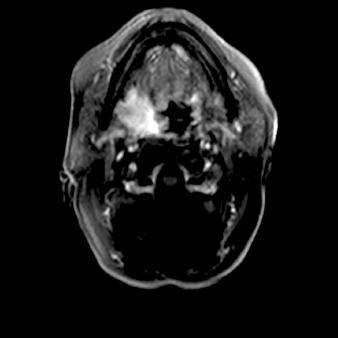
Contrast enhanced magnetic resonance imaging (MRI) before cryoablation – 10 cm in length tumour invading parapharyngeal, submandibular, palatoglossal arch and palatopharyngeus.
